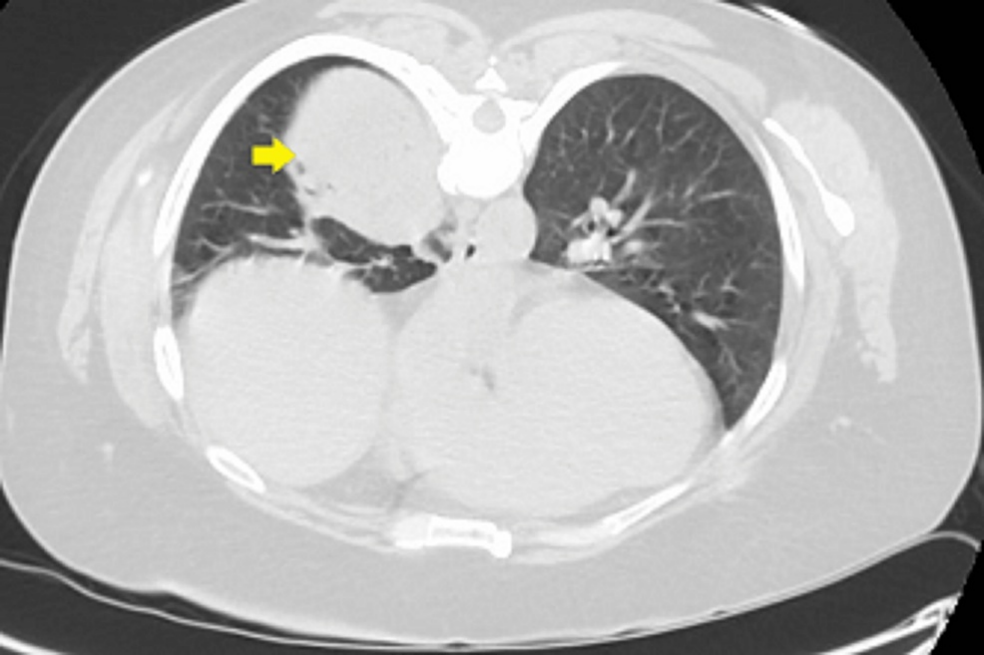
CT of the chest showing 5 x 3 x 5 cm lung mass abutting pleura (arrow).
